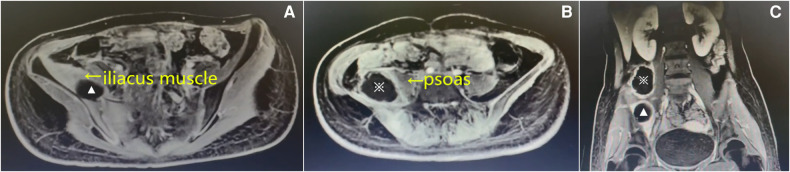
Enhanced magnetic resonance imaging (MRI) on the 3rd admission day. Axial (A) and (B) and coronal T1-weighted images (C) showing that the abscesses were located in the right iliacus muscle (▴) and posterolateral to the right psoas (※).
